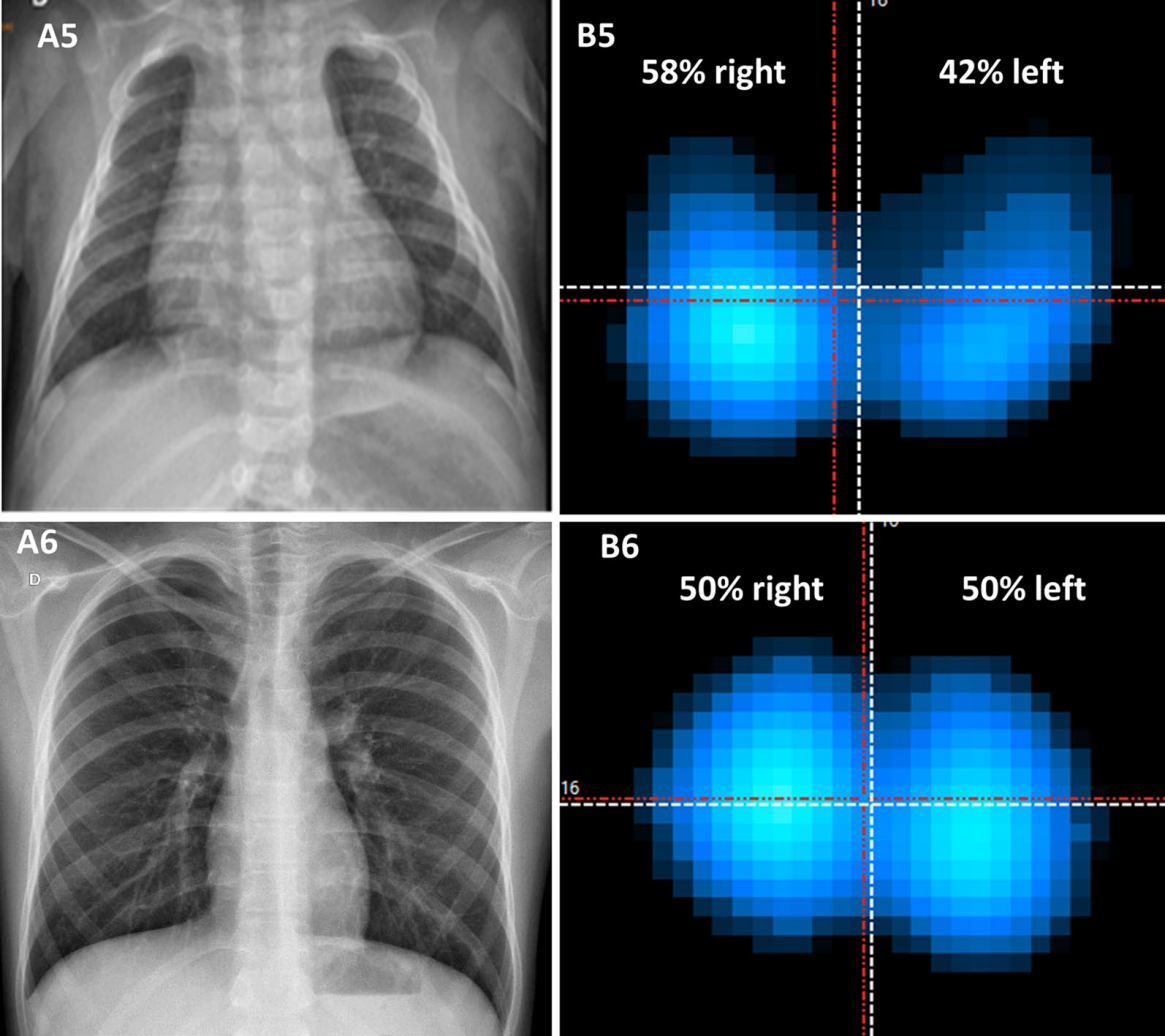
Chest radiograph images (A) and EIT images (B) of patient 5 and patient 6. The patient 5 was a three months-old girl and the patient 6 an 11 years-old boy. The radiograph images of both patients were normal, and EIT also showed no changes in ventilation distribution. The color scale of the EIT corresponds to the tidal volume variation present at the different ROIs: dark blue corresponds to a lower ventilation variation, and white corresponds to high tidal volumes
